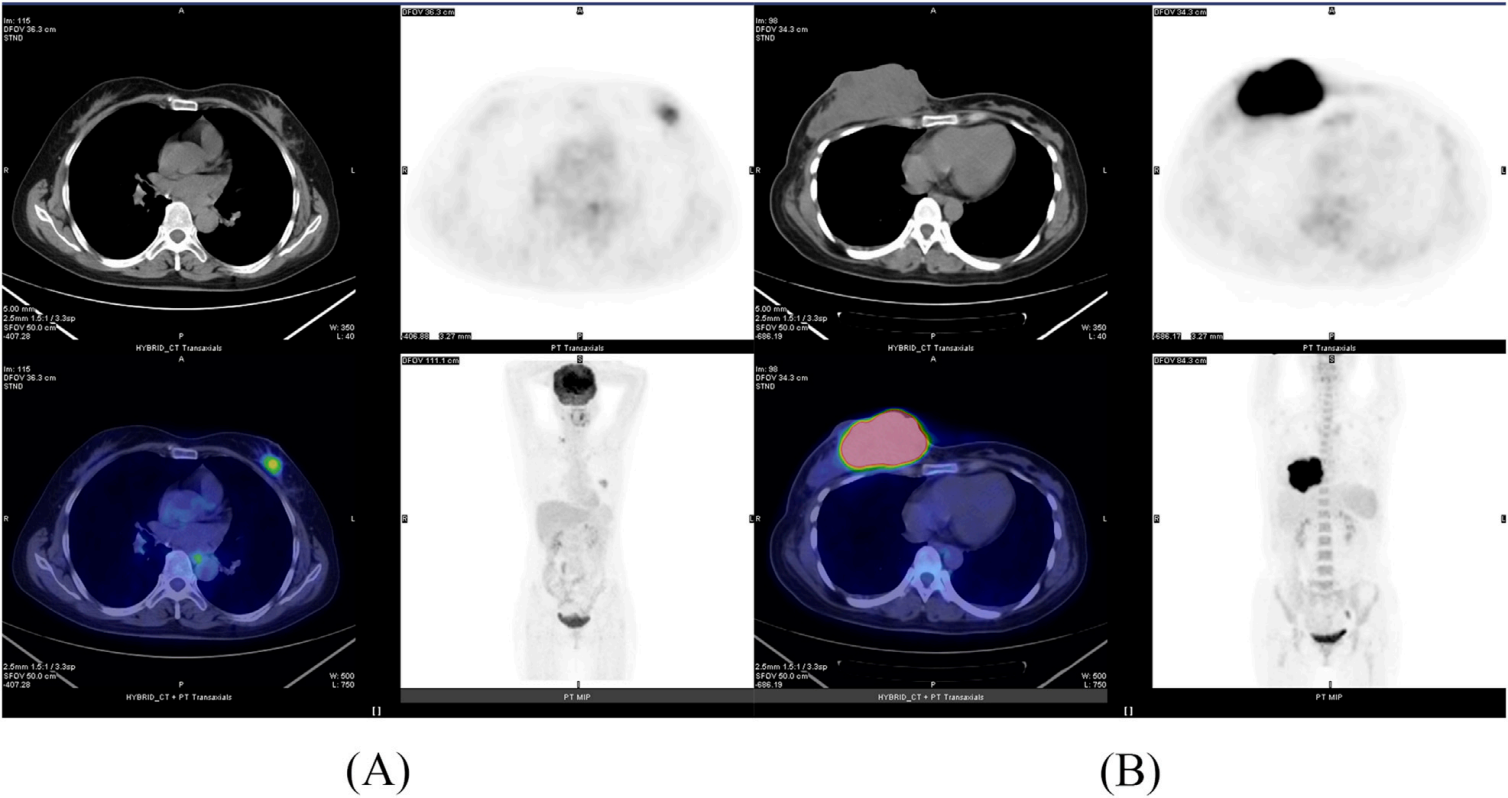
The ^18^F-FDG PET/CT images of two patients. (A) Patient A: SUV_max_ = 4.60, SUV_mean_ = 3.28, SUV_peak_ = 3.42. (B) Patient B: SUV_max_ = 28.55, SUV_mean_ = 10.99, SUV_peak_ = 24.22.
